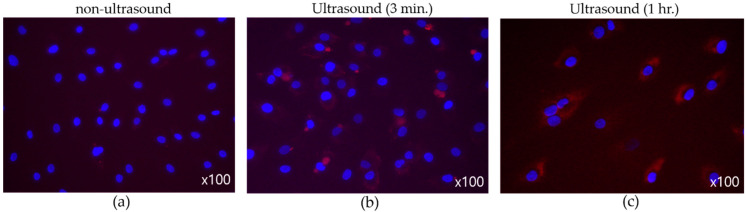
(Flat tip, 40 V_pp_) Effect of ultrasound on membrane permeability. (a) No sonification, (b) three-minute exposure, (c) one-hour exposure.
